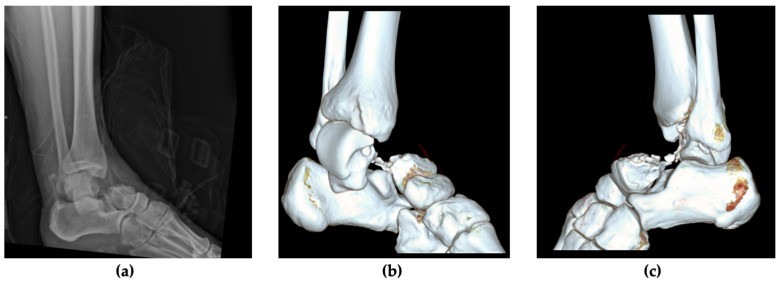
Radiological findings after immediate patient presentation in the vacuum splint. (a) Lateral view of the left ankle, showing a talar neck fracture with tibiotalar and subtalar dislocation; preoperative CT scans with 3D reconstruction: (b) medial view showing an extruded body of the talus; (c) lateral view showing a comminution zone at the lateral talar neck.
